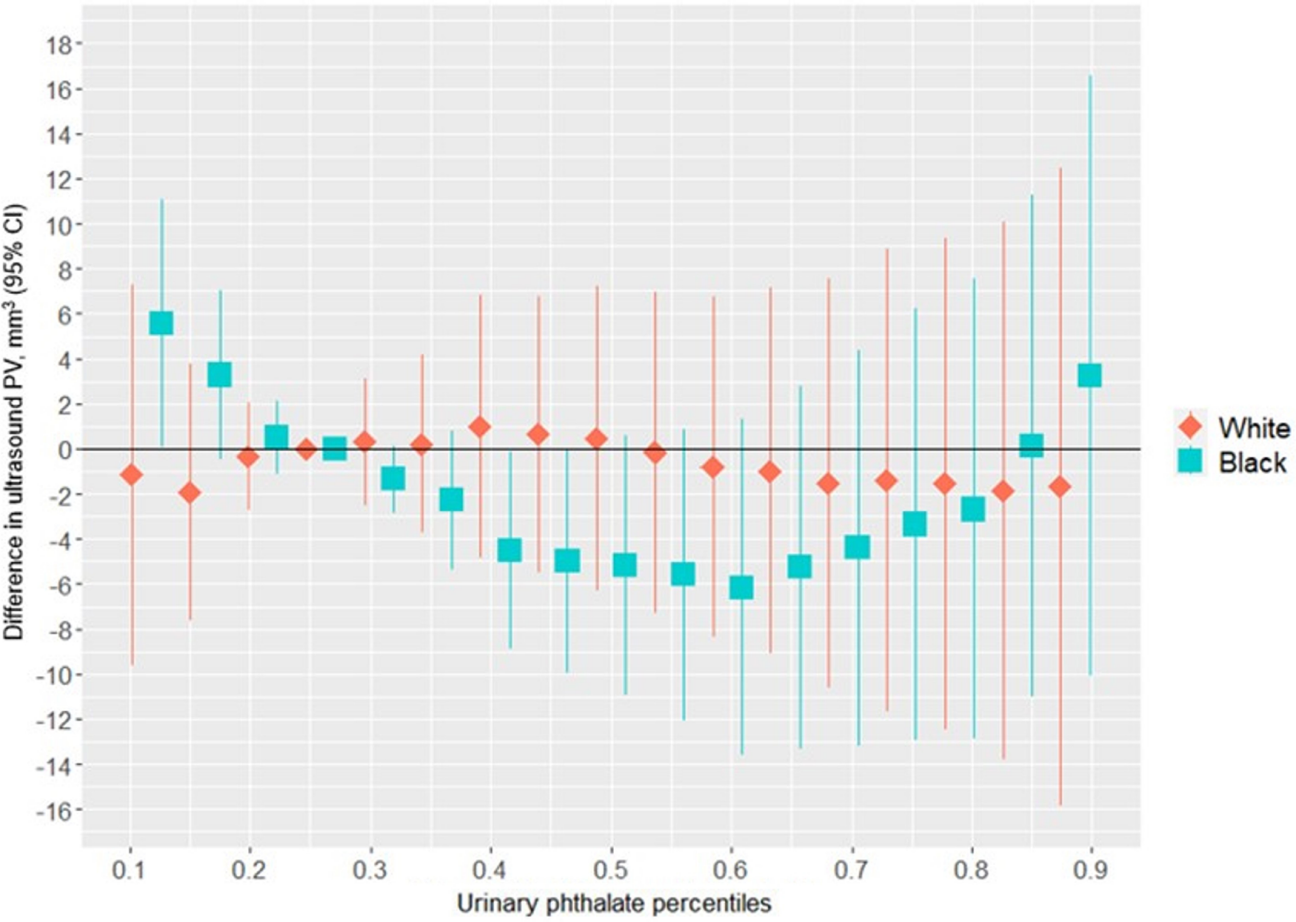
Differences in ultrasound PV associated with percentiles of a urinary phthalate metabolites mixture (with the 25th percentile as the reference), adjusted for maternal specific gravity, maternal age, maternal BMI, maternal cigarette smoke, maternal education level, and gestational age at enrollment, among male infants born to women in Charleston, South Carolina, by racial identity (*n* = 178).
